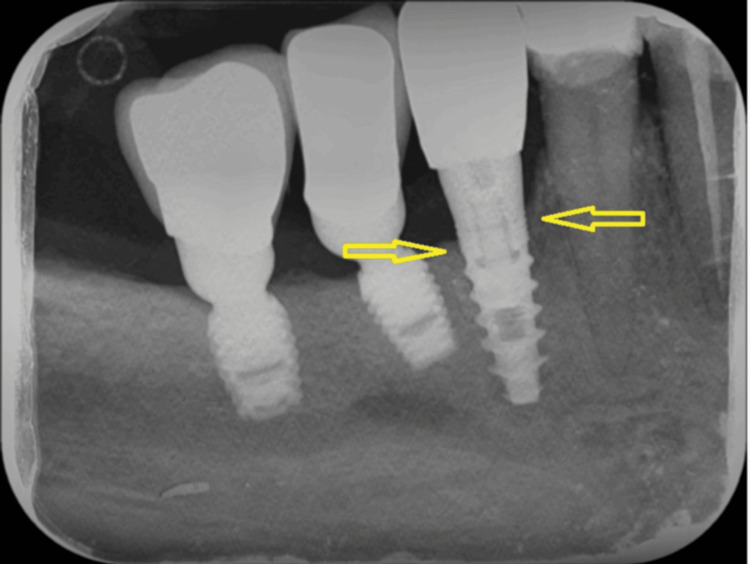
Control X-ray three months after prosthetic restoration. The new bone is rebuilt and stable
